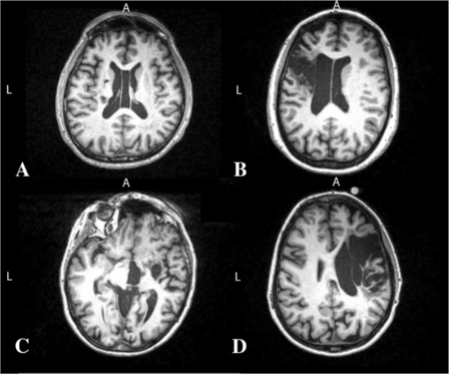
A Comparison Across Different Stroke Lesion Profiles at Maximum Lesion. MRI scans of individuals who sustained a subcortical left-hemisphere stroke, b cortical left-hemisphere stroke, c subcortical right-hemisphere stroke, d cortical right-hemisphere stroke
